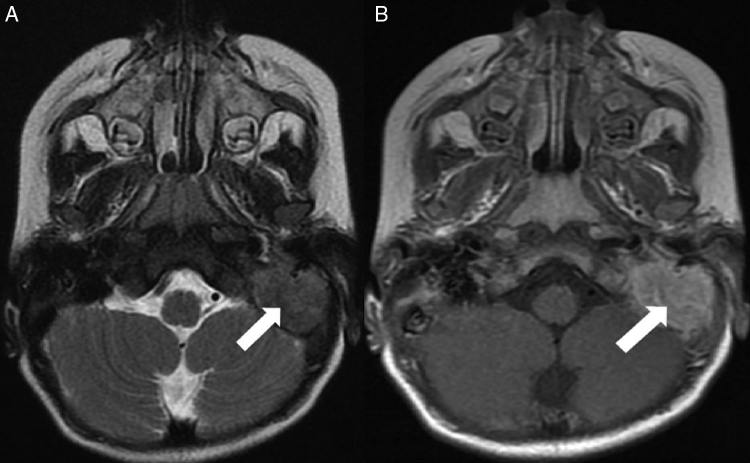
Axial T2-weighted magnetic resonance image (A) demonstrates a hypointense mass (arrow) occupying the left mastoid cavity. Postcontrast T1-weighted image; (B) reveals homogeneous enhancement within the mass (arrow).
